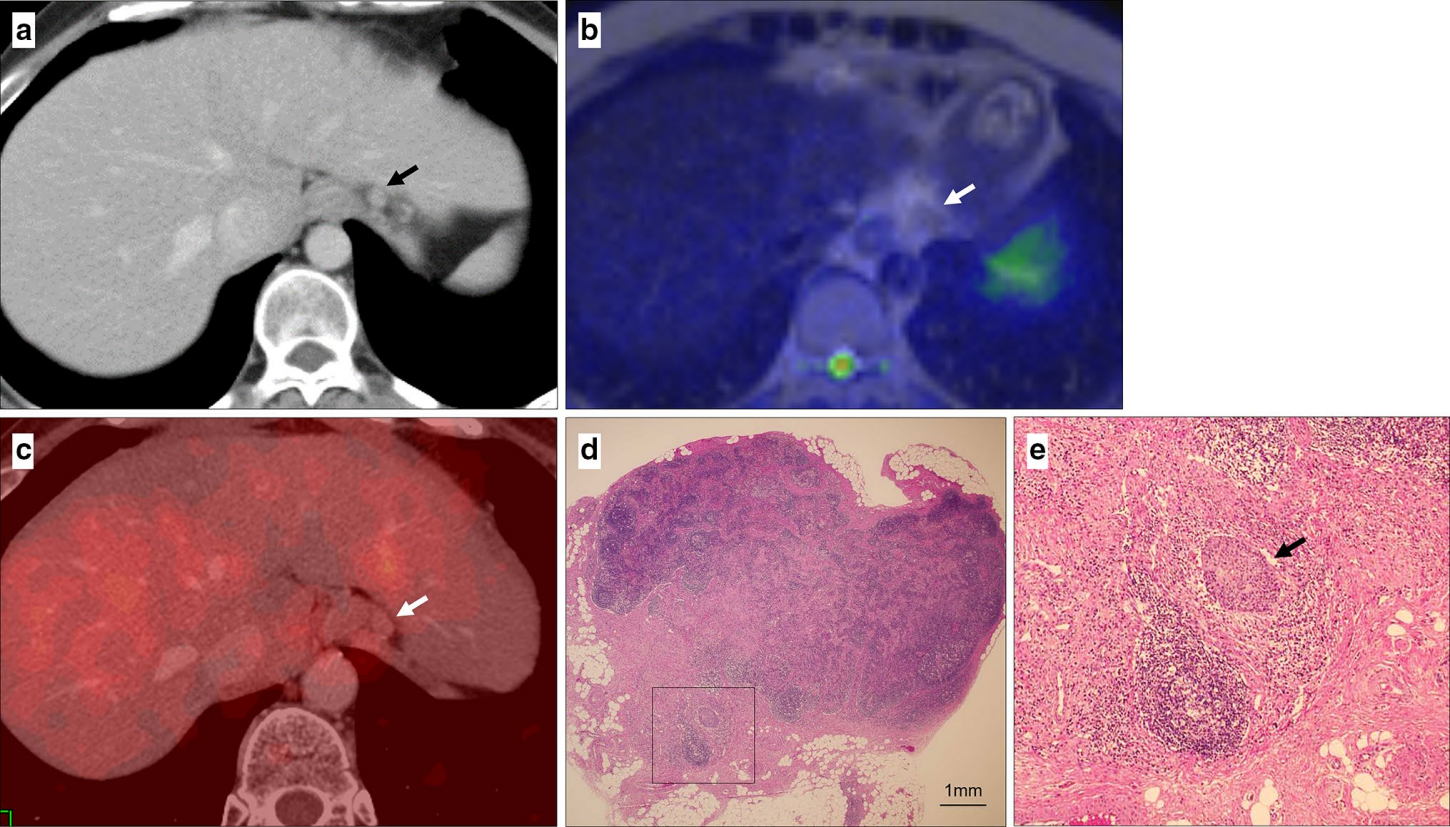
False negative lymph node of 10 mm in size by DWI and PET (arrow). a Paracardial lymph node was detected on contrast-enhanced CT as metastasis. It was hypointense on DWI (b) and hypoaccumulate on PET (c). d, e The node was identified as metastatic with micrometastasis (d square, e arrow)
